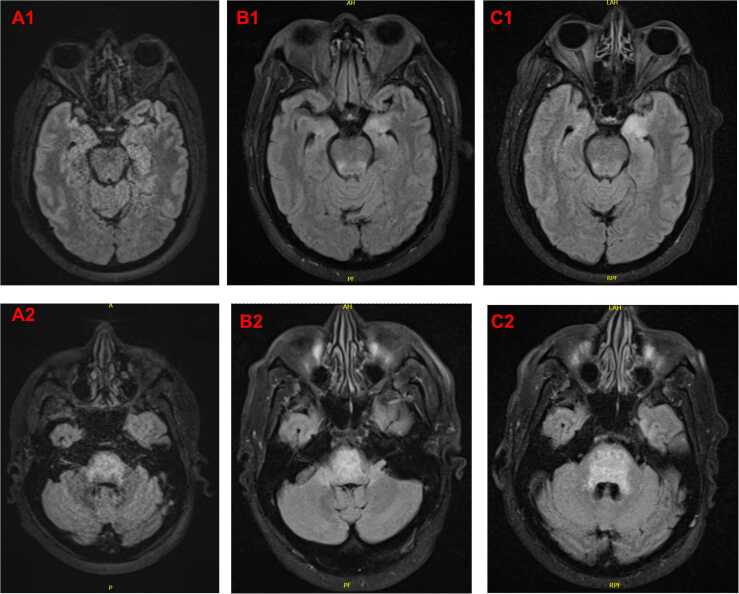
Magnetic resonance brain imaging of a patient with herpes simplex virus rhombencephalitis. Image set A are T2 sequence images obtained on hospital day 1. Image set B are FLAIR sequences obtained on hospital day 5. Image set C are FLAIR sequences obtained on hospital day 8. Images labeled with “1” are cuts through the hippocampi and images labeled with “2” are cuts through the pons at the infraorbital level.
